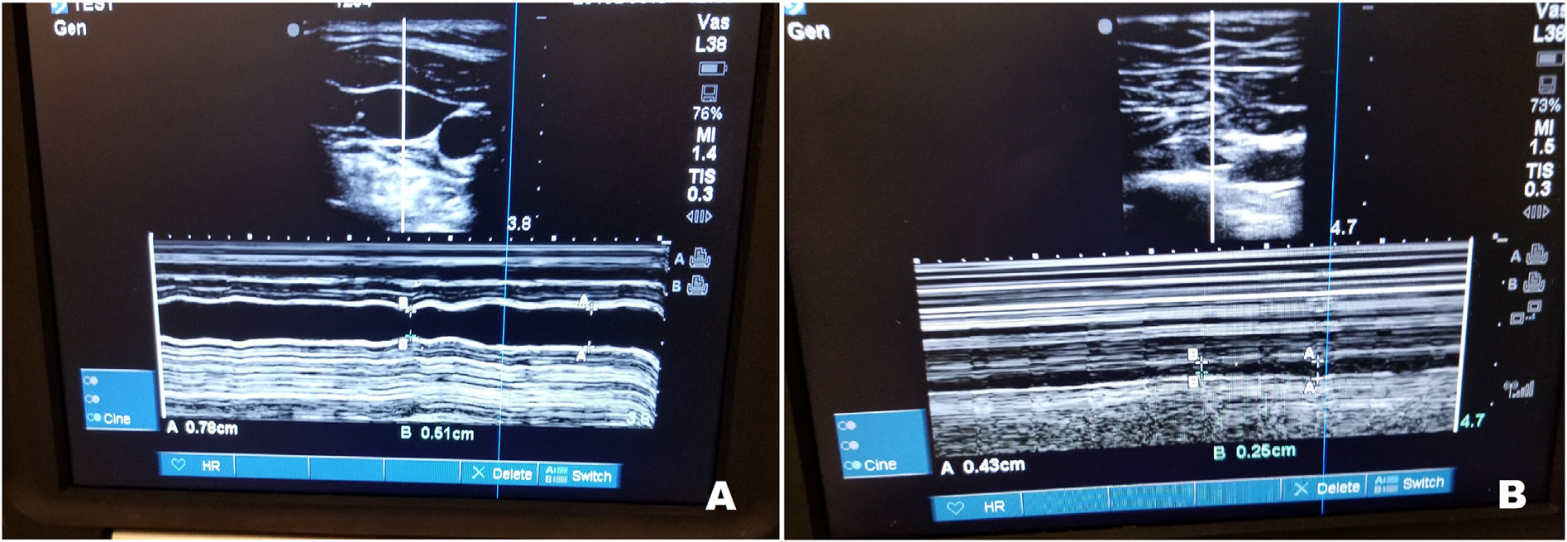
AM-mode ultrasound of the internal jugular vein, 1 B: M-mode ultrasound of the subclavian vein.
